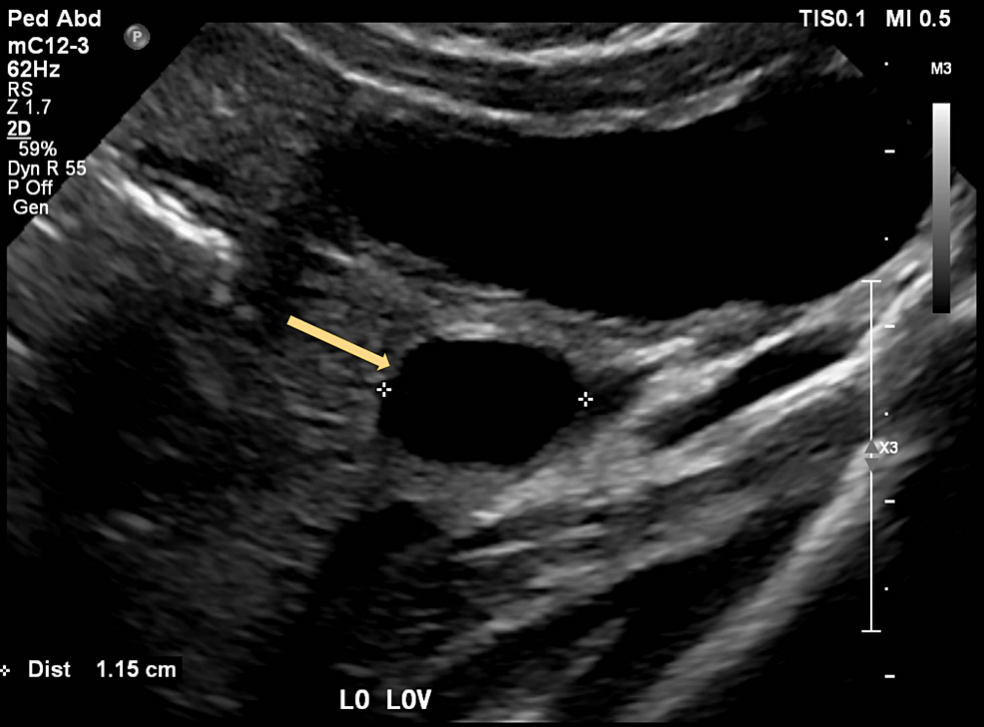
Ultrasound of left ovarian cyst
